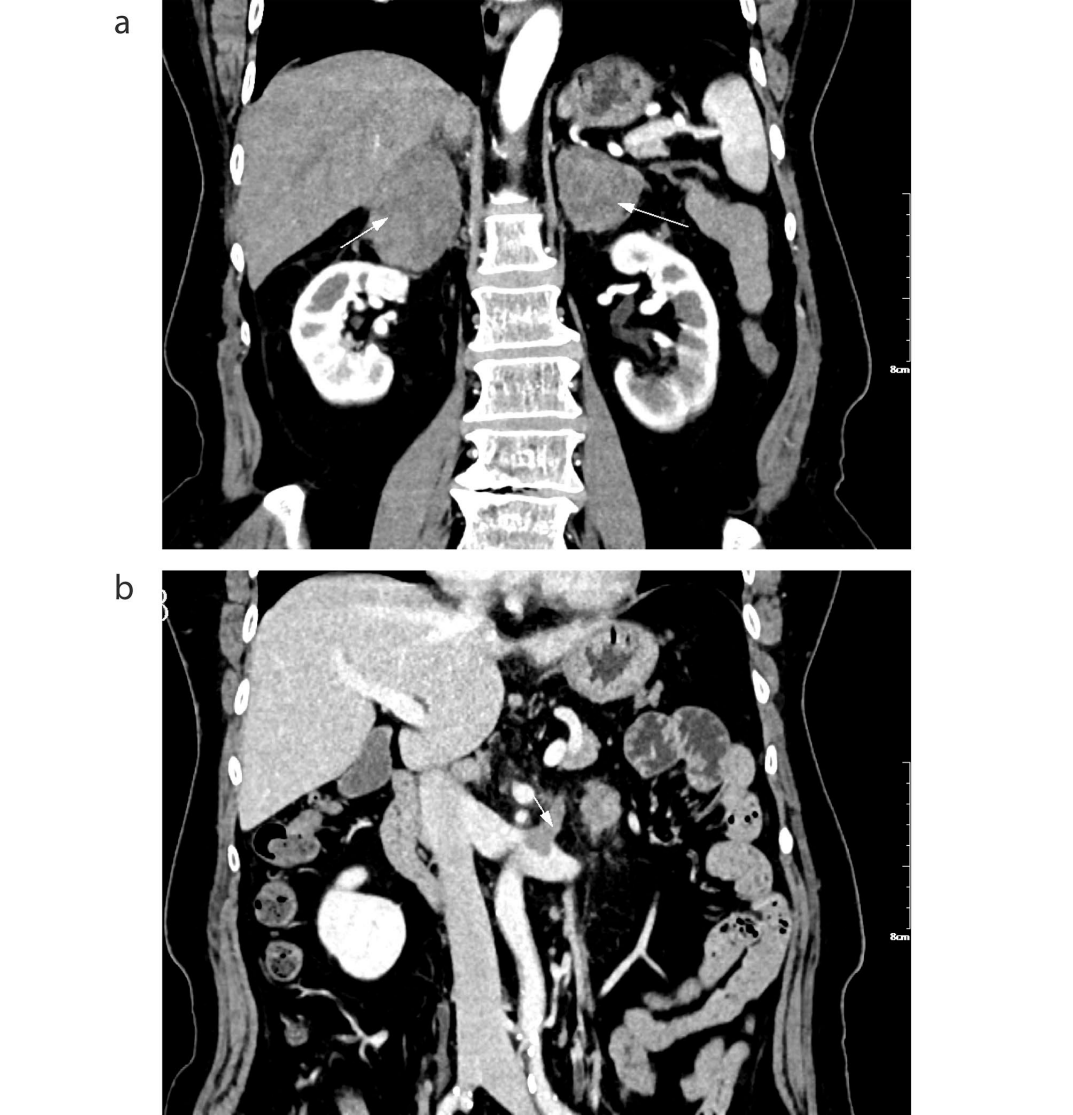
(A, B) Contrast-enhanced spiral computed tomography (CT) showed mild to moderate enhancement of bilateral masses and central adrenal vein tumor thrombus(arrow)
